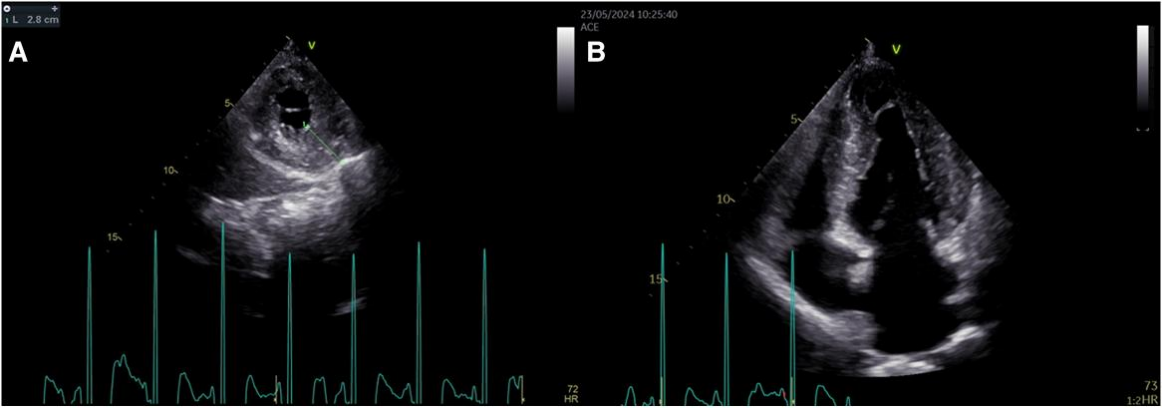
In (*A*), the parasternal short-axis view shows a maximum wall thickness of 28 mm in the apical region of the left ventricle. In (*B*), the apical four-chamber view demonstrates marked left ventricular hypertrophy.
